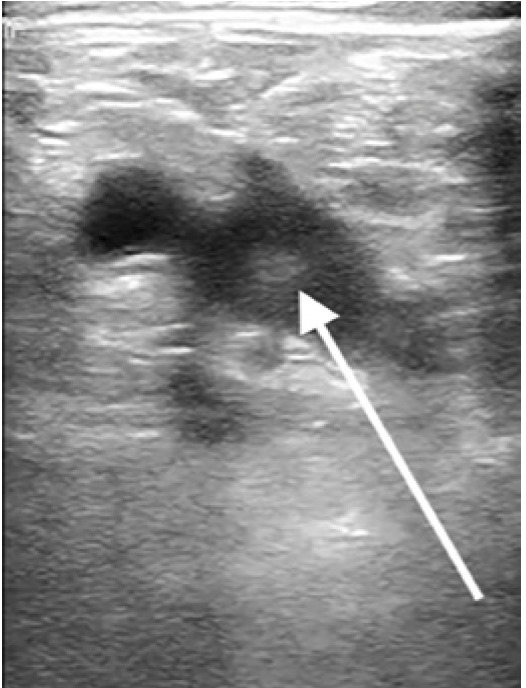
Point-of-care ultrasound of the lower extremity with arrow identifying hyperechoic turbulent flow in the common femoral vein.
